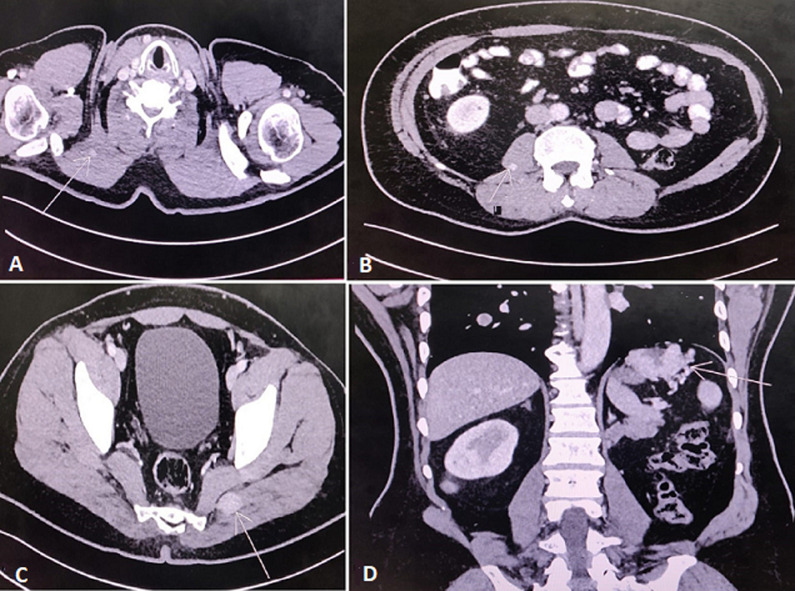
A) CT scan showing metastasis to the right trapezius muscle (arrow); B) metastasis to the right psoas (arrow); C) metastasis to left gluteus medius (arrow); D) metastasis to left hemidiaphragm (arrow)
